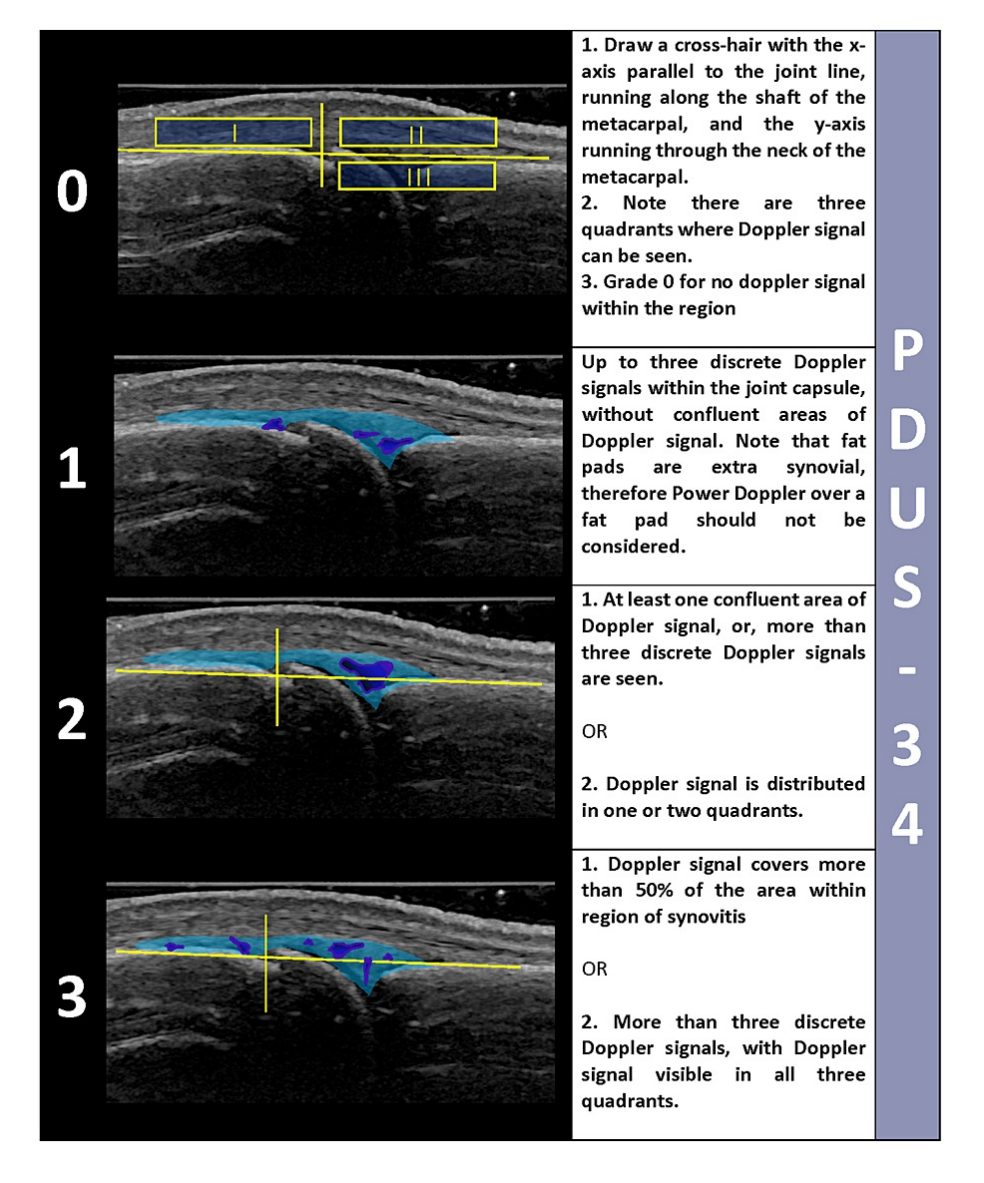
Example of scoring rules created to improve reliability This figure provides a sample of the 50% rule and the quadrant rule power Doppler scoring system for metacarpophalangeal joint (MCP) dorsal long view. The 50% rule traditionally differentiates between grade 2 and grade 3 scores. However, the 50% rule is sometimes difficult to apply in all situations; thus, the quadrant rule was developed to assist in these difficult-to-score circumstances. Power Doppler scores 0-3 are defined utilizing both rules.
